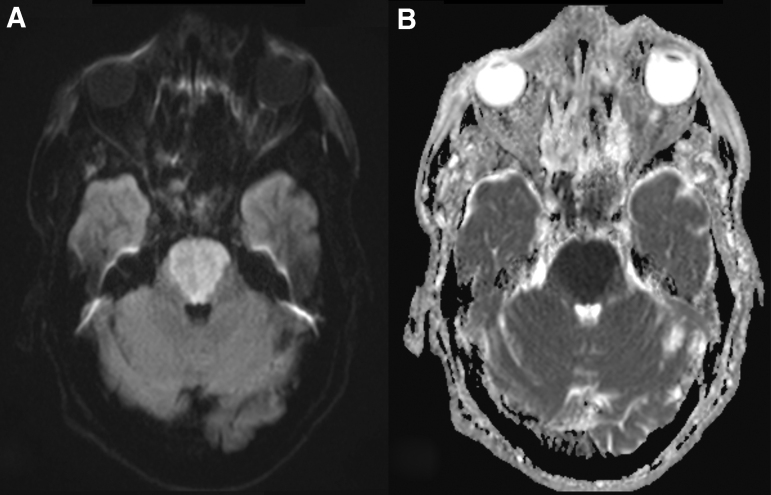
Axial magnetic resonance images. (A) Diffusion-weighted image (DWI) confirms acute pontine ischemia with (B) corresponding apparent diffusion coefficient (ADC) image.
